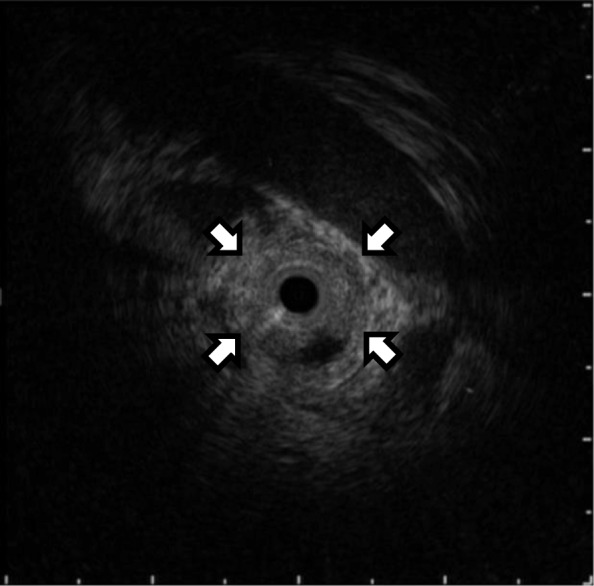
Intraductal ultrasonography (IDUS) findings. Intraductal ultrasonography (IDUS) suggested the tumor (arrow) was located in the bile duct wall
